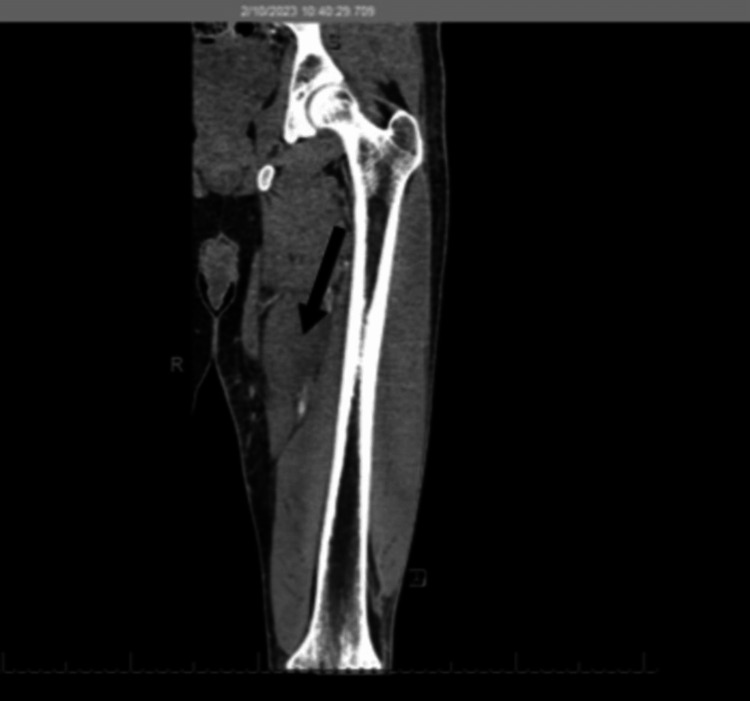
Coronal CT of the left lower extremity showing hypoattenuation of the adductor longus muscle within the medial compartment and associated subcutaneous edema (arrow)
